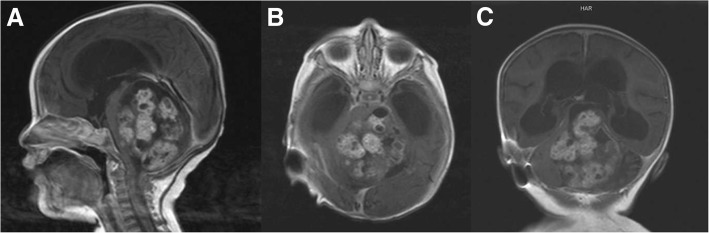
Preoperative T1-weighted a sagittal, b axial, and c coronal MRI scans demonstrating a 70 × 60 × 55 mm heterogeneously enhancing posterior fossa tumor with marked brain stem compression and obstructive hydrocephalus
